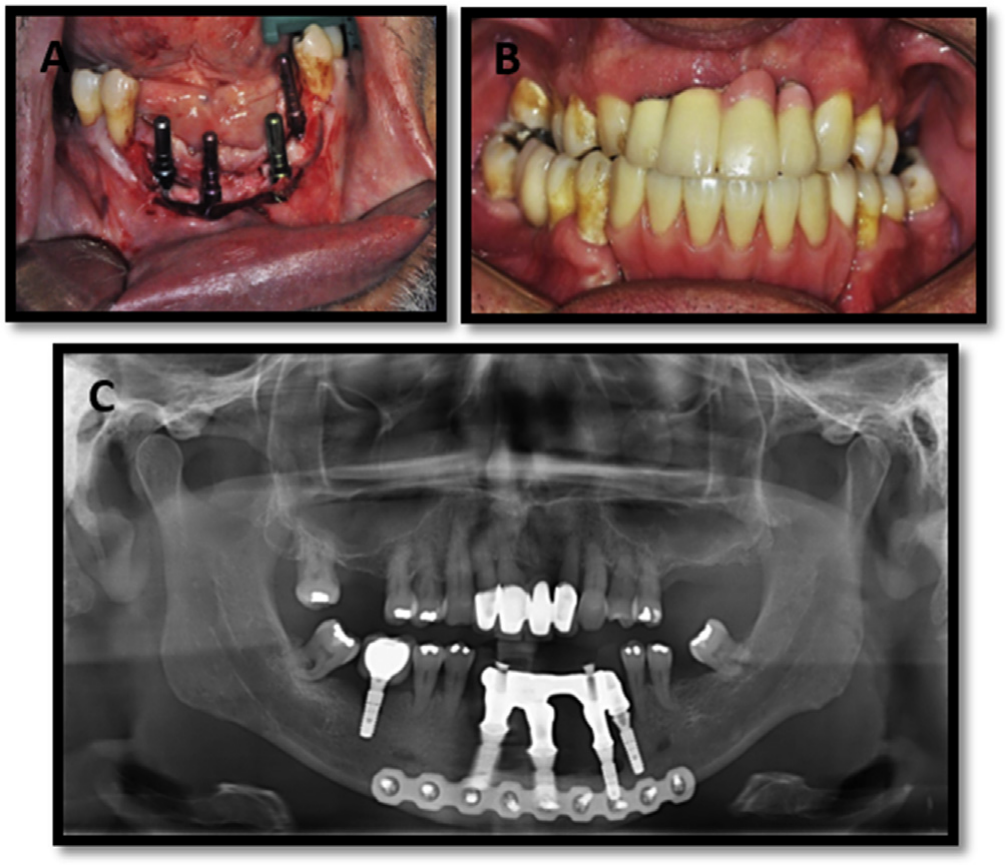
A. Rehabilitation with 4 implants, 8 months after resection surgery. B and C. Clinical and radiographic aspects after rehabilitation with implant-supported prosthesis.
